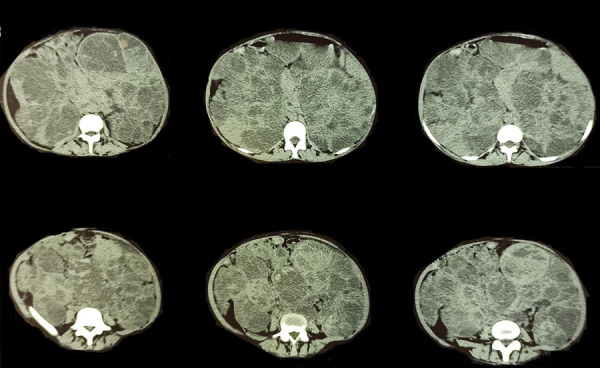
Axial CT scan showed two large kidneys with budding and heterogeneous masses with mixed matrix (tissue, fluid, and fat).
